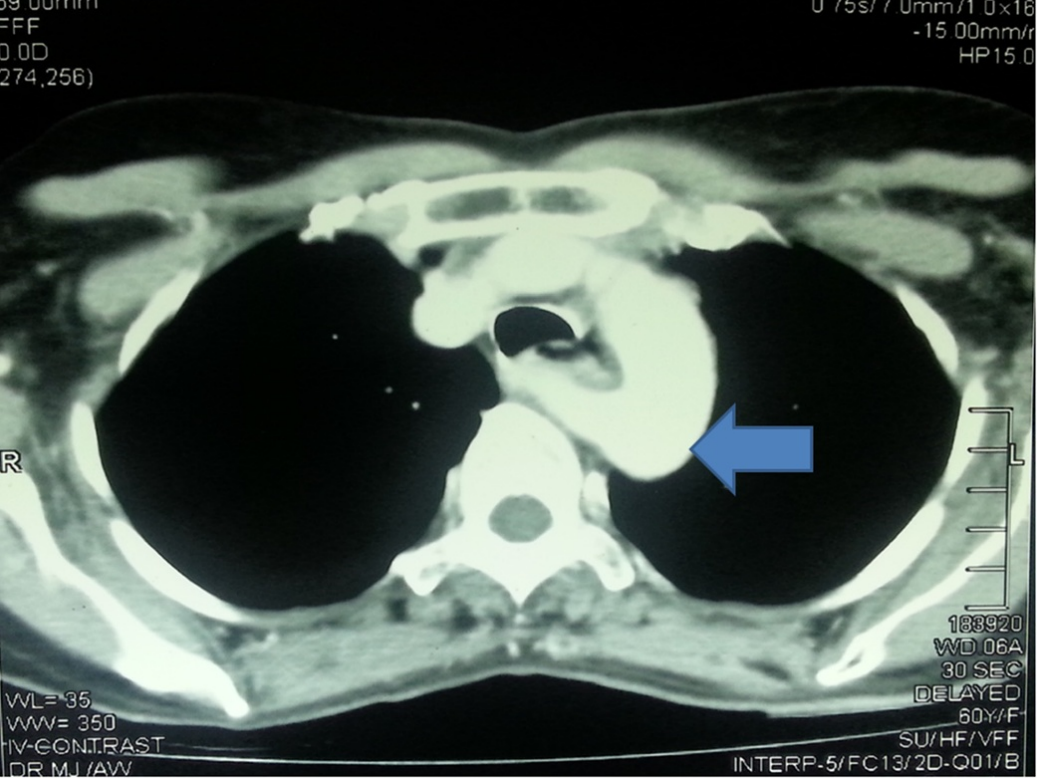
Contrast enhaced CT thorax showing the course of aberrant R/subcalvian artery behind the oesophagus (blue arrow shows the aberrant vessel).
